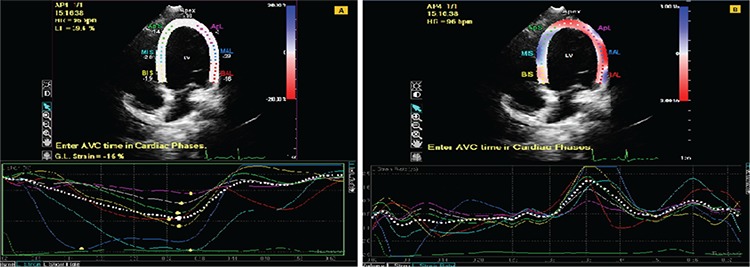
Two dimensional strain and strain rate analysis through speckle tracking echocardiography imaging of euthyroid Hashimoto’s thyroiditis. A) Strain analysis, B) strain rate analysis
